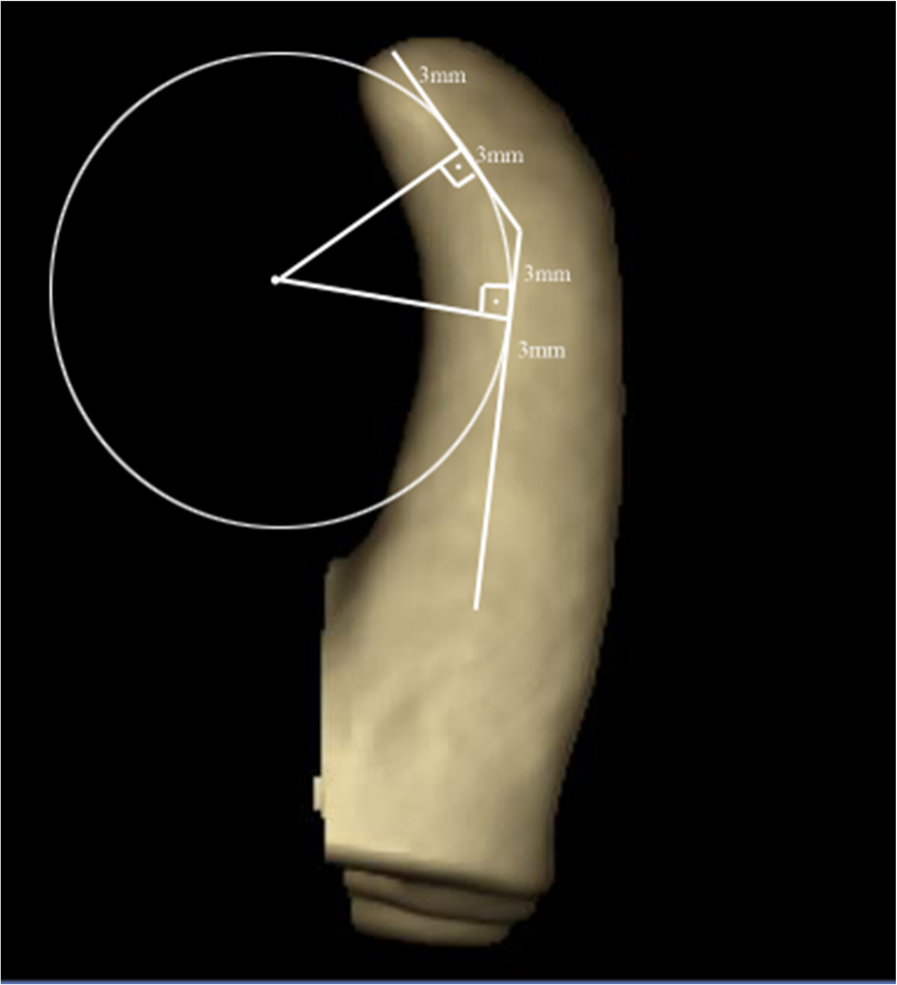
Three-dimensional CBCT images showing the measurement of root curvature.
